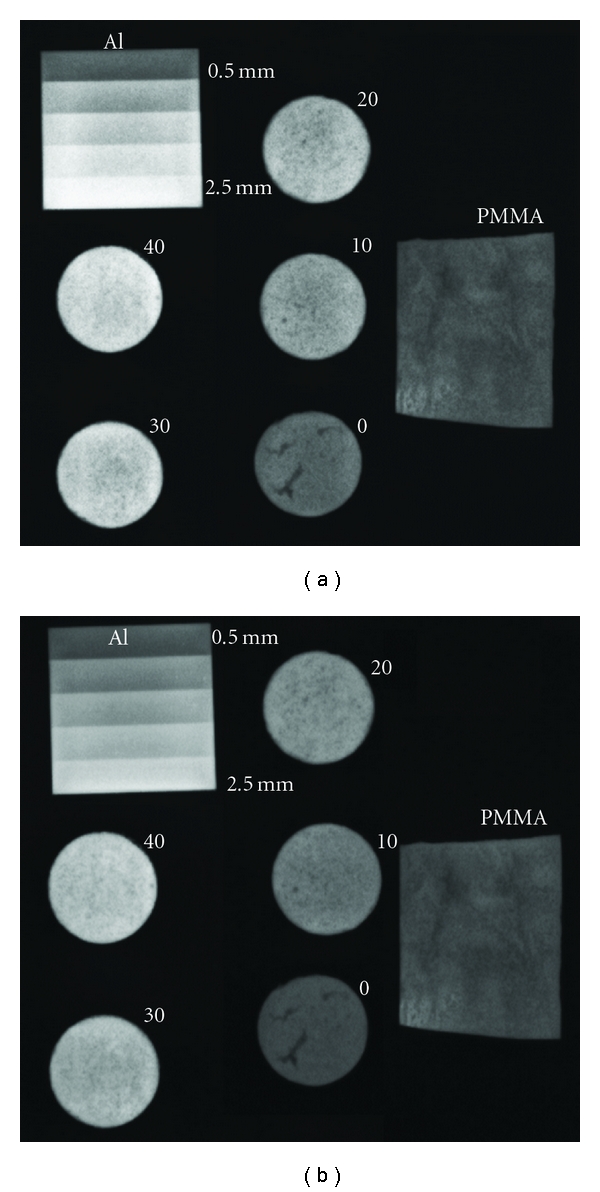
X-ray image comparing pCPC samples containing 0 to 40% ZrO_2_, with a commercial PMMA cement (Vertebroplastic) and an aluminium wedge with 0.5 to 2.5 mm in thickness. The X-ray opacity was measured at 1 mAs, with 40 kV (a) and 80 kV (b).
